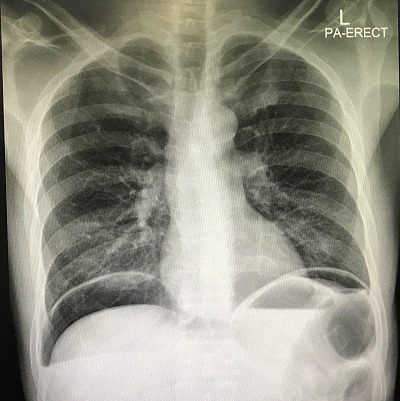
First X-ray was in ED which showed pneumoperitoneum with air fluid line
